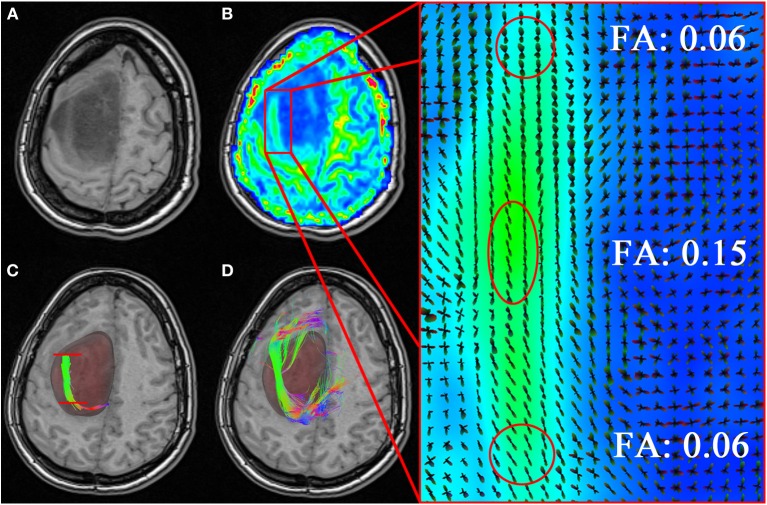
Neurosurgical application of the MultiPeak-RTT method. (A) T1-weighted image revealing an anaplastic astrocytoma tumor extending across the left hemisphere of the brain. (B) Coherent structure within the tumor region in accordance to local orientations (zoomed rectangle). In (C) one can observe a premature termination of the fiber tracts inside the tumor (red lines). By lowering the FA threshold to 0.06, we allow the tracts to momentarily step over small local changes in the diffusivity and continue their course toward the cortex (D). (TP dataset).
